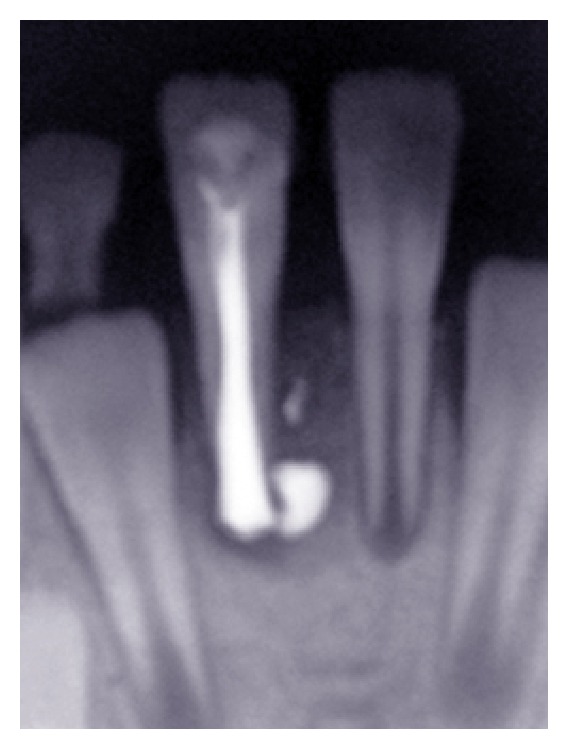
One month follow-up radiograph.
